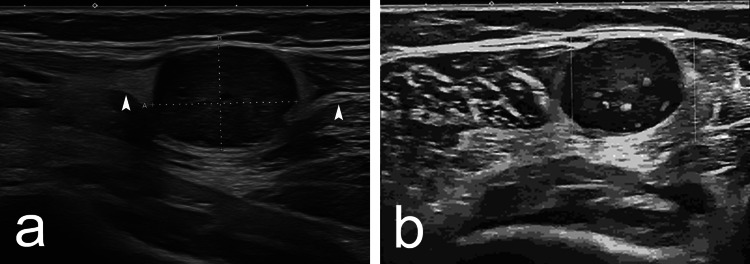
Ultrasound examination presenting a well-defined, hypoechoic, encapsulated mass with internal heterogeneity (a). Doppler ultrasound examination presenting a well-defined, nonvascular, encapsulated structure (b). The white arrowhead shows the medial sural cutaneous nerve.
